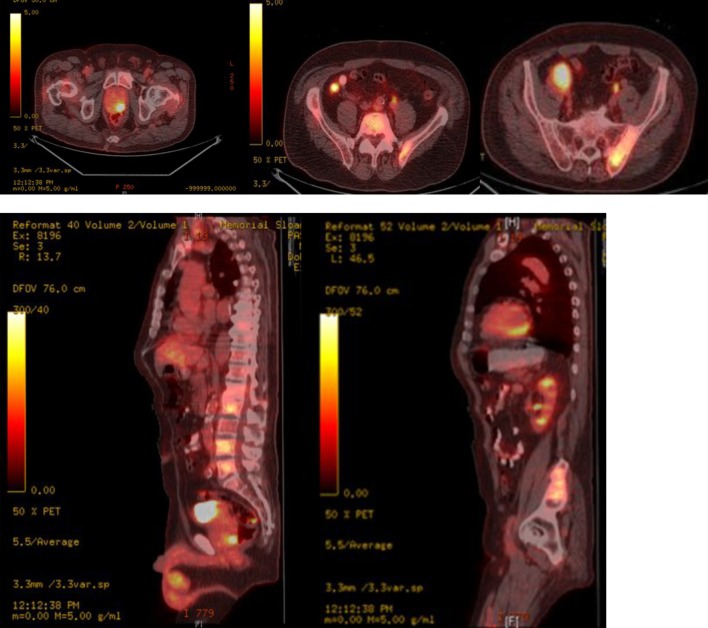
Whole body PET-CT showing FDG avid uptake in posterior lobe of prostate, axial spine, iliac bones and bilateral iliac lymph nodes.
